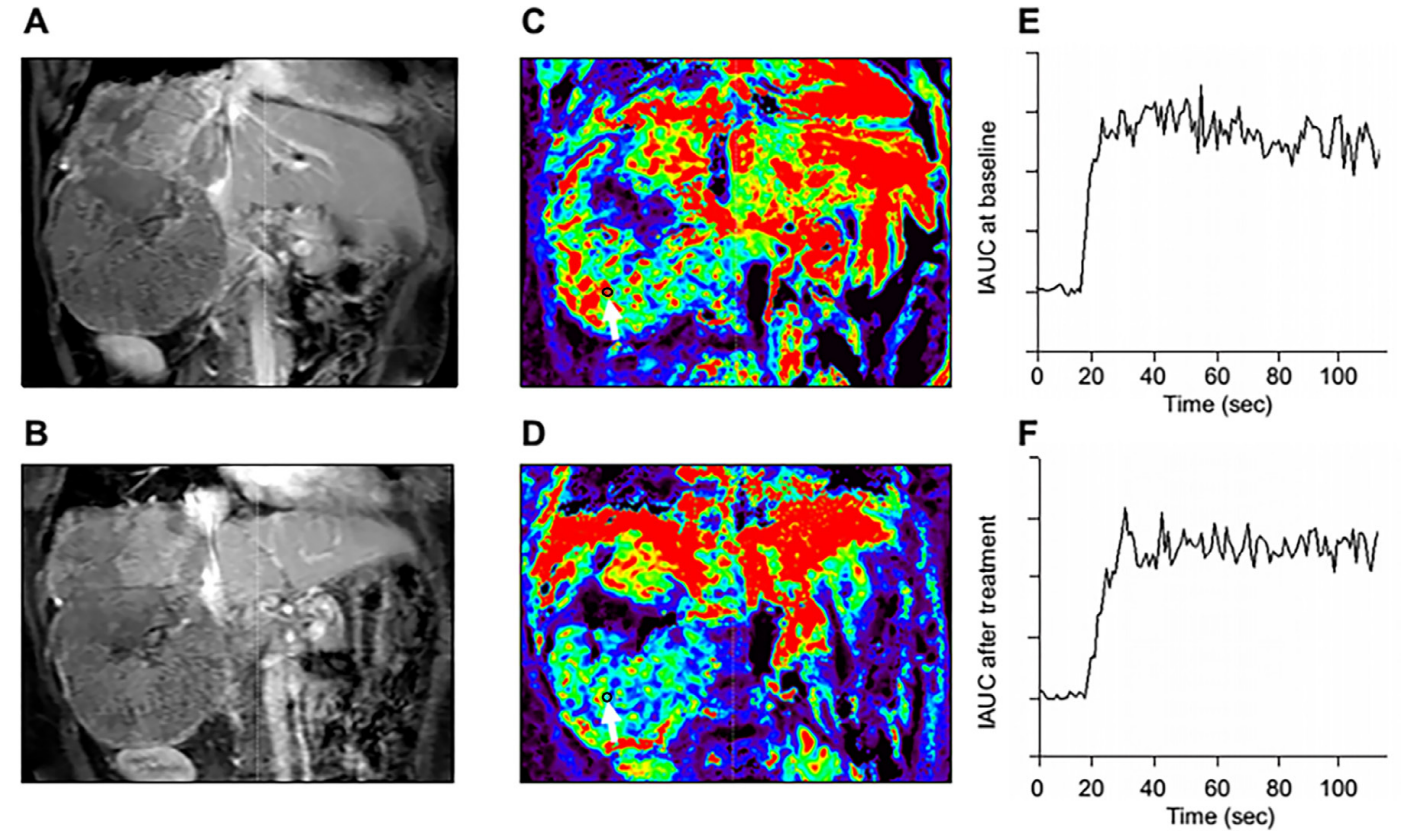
Representative DCE-MRI data in one advanced HCC patient A. Post-contrast T1-weighted MRI at baseline and B. after 14 days of study treatment. Color K^trans^ map of the tumors was greatly heterogeneous due to tumor necrosis and ROI was selected in the most enhanced tumor region. C. Corresponding color K^trans^ maps at baseline and D. after 14 days of study treatment. Hypervascular area is shown in red. The selected ROI (black cycle ) for K^trans^ measurement is indicated by white arrows, In this patient, the K^trans^ values at baseline and after study treatment were 798.6×10-3/min and 206.6 × 10-3/min, respectively. E. The initial area under the gadolinium concentration-time curves (IAUC) at baseline F. and after study treatment from the same patient. The IAUC values at baseline and after study treatment were 1526.2 mmol/kg×s and 1376.1 mmol/kg×s, respectively.
